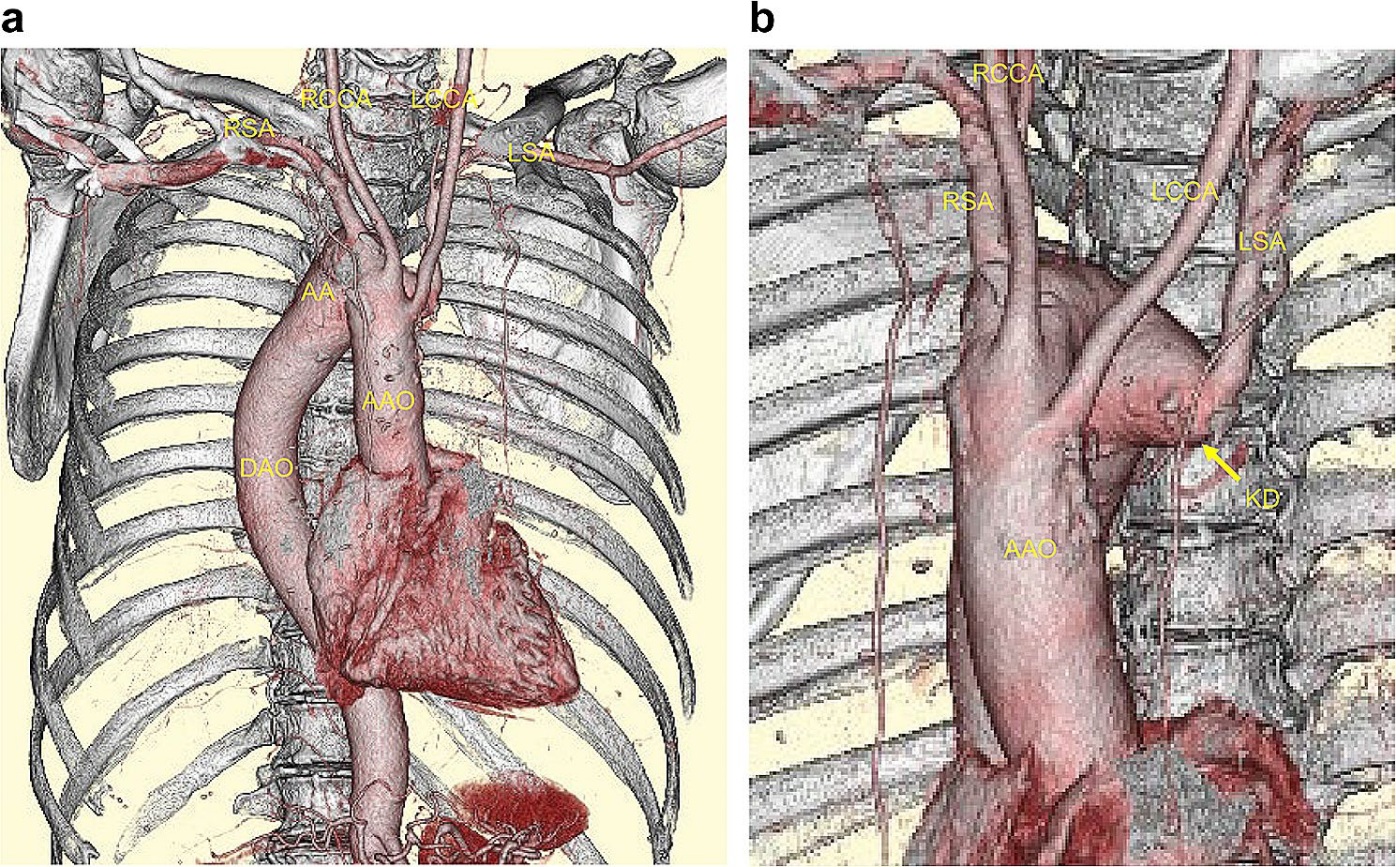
Three-dimensional computed tomography scan image of the right aortic arch (a) The anterior view shows the right aortic arch and the right descending aorta running on the right side of the vertebral body (b) The lateral view shows the so-called Kommerell diverticulum of the left subclavian artery AAO, ascending aorta; DAO, descending aorta; LCCA, left common carotid artery; LSA, left subclavian artery; RCCA, right common carotid artery; RSA, right subclavian artery; KD, Kommerell diverticulum
